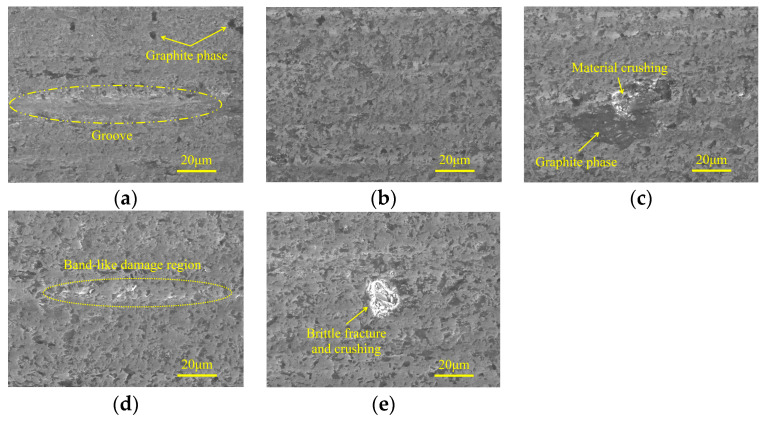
Micro-morphologies under different ultrasonic amplitudes in finishing grinding stage: (a) $T_{A}$: 0 μm $W_{A}$: 4 μm; (b) $T_{A}$: 4 μm $W_{A}$: 4 μm; (c) $T_{A}$: 8 μm $W_{A}$: 4 μm; (d) $W_{A}$: 0 μm $T_{A}$: 4 μm; and (e) $W_{A}$: 8 μm $T_{A}$: 4 μm.
